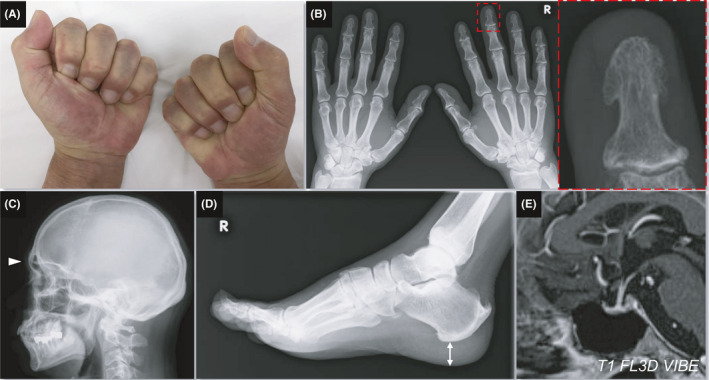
A, Positive fist sign. B, Cauliflower‐like tufting of the distal phalanxes in a hand X‐ray. The dotted line in the left panel corresponds to the outline of the right panel. C, Head X‐ray showed enlargement of the frontal sinus (*arrowhead*). D, Foot X‐ray showed heel pad thickness of 22 mm (*two‐way arrow*). E, Contrast‐enhanced pituitary magnetic resonance imaging showed no tumor
